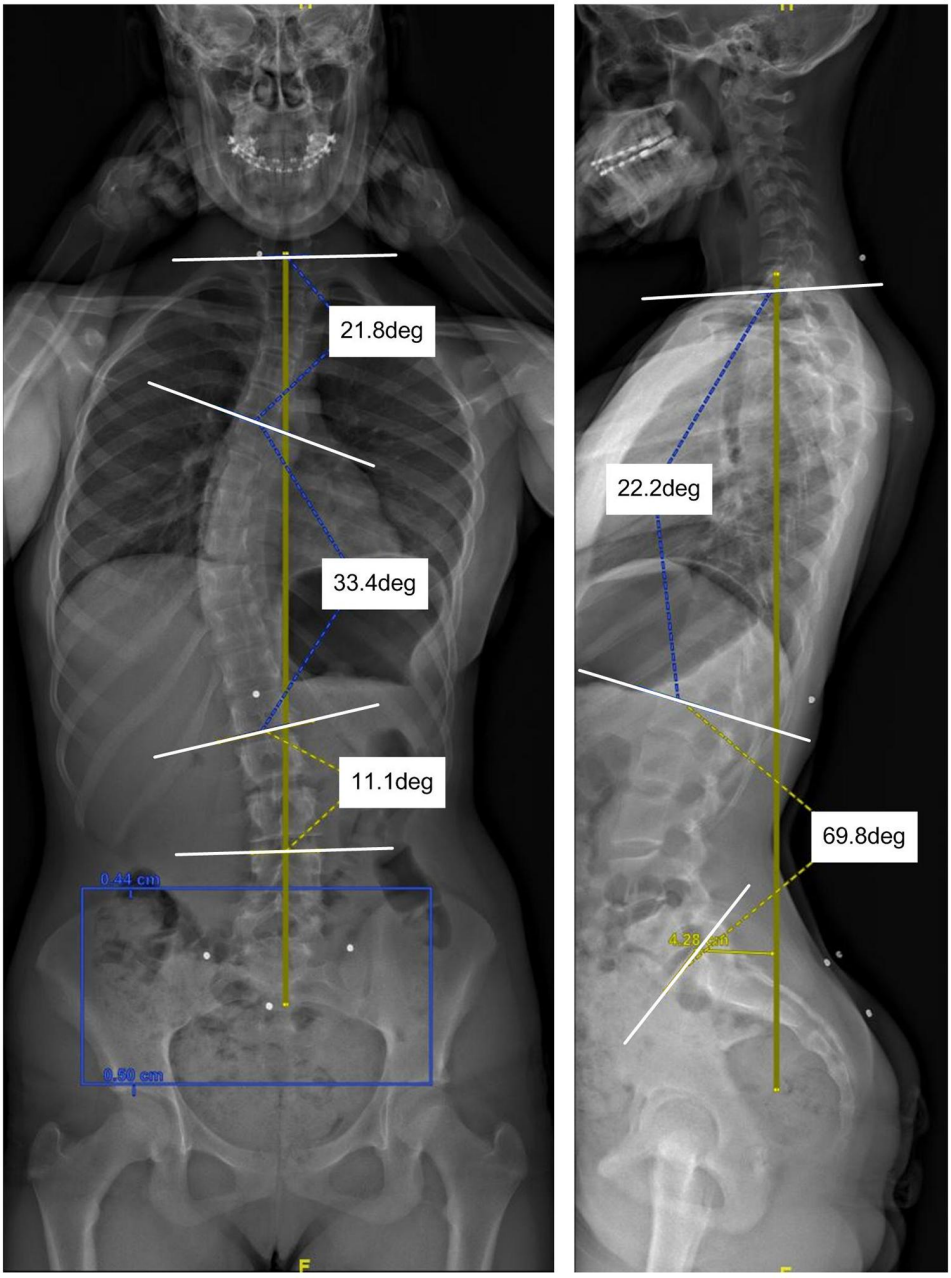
X-ray image of an AIS subject with basic radiographic measurements – 3 cobb angle measurements.
